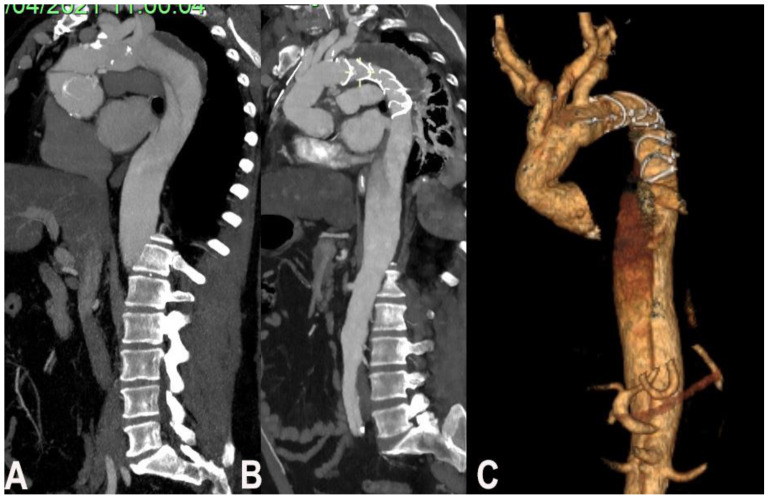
(A). Chronic residual aortic dissection, (B) post-operative CT scan, (C) post-operative CT scan reconstruction.
